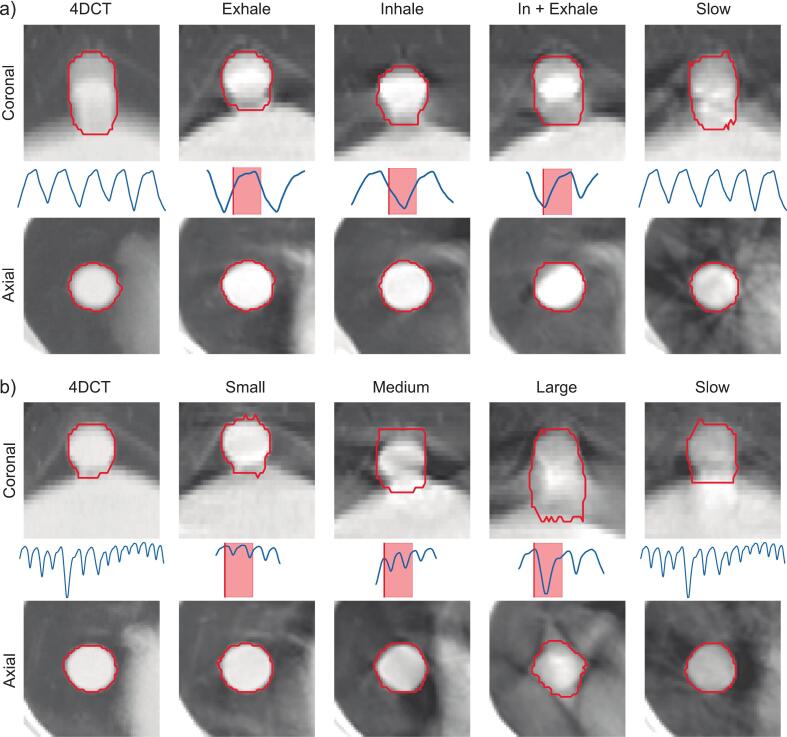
Images and manual contours on the average 4DCT reconstruction, three 6-second HS-CBCT scans, as well as the 60-second HS-CBCT scan (Slow) of the slow (a) and irregular (b) breathing trace. The 6-second HS-CBCT scans were timed at different timestamps (shaded red area) corresponding to Exhale-only, Inhale-only & Inhale-to-exhale for slow breathing (a) and Small, Medium & Large magnitude of breathing motion for irregular breathing (b). The images and contours are displayed in the axial and coronal view through the center-of-mass of the contoured tumor. The traces indicate the input provided to the lung compression system and indicate the extent of lung compression. (For interpretation of the references to colour in this figure legend, the reader is referred to the web version of this article.)
